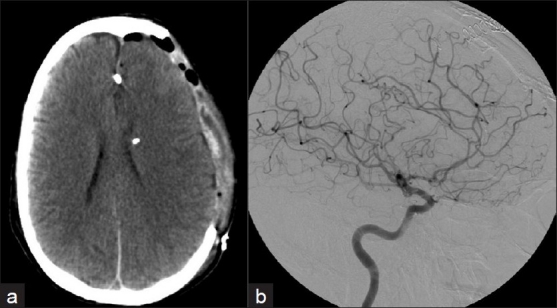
a) CT scan following aneurysm clipping through a frontal transcallosal approach; b) conventional cerebral angiogram through the left internal carotid artery shows complete aneurysm obliteration with preservation of the parent artery
